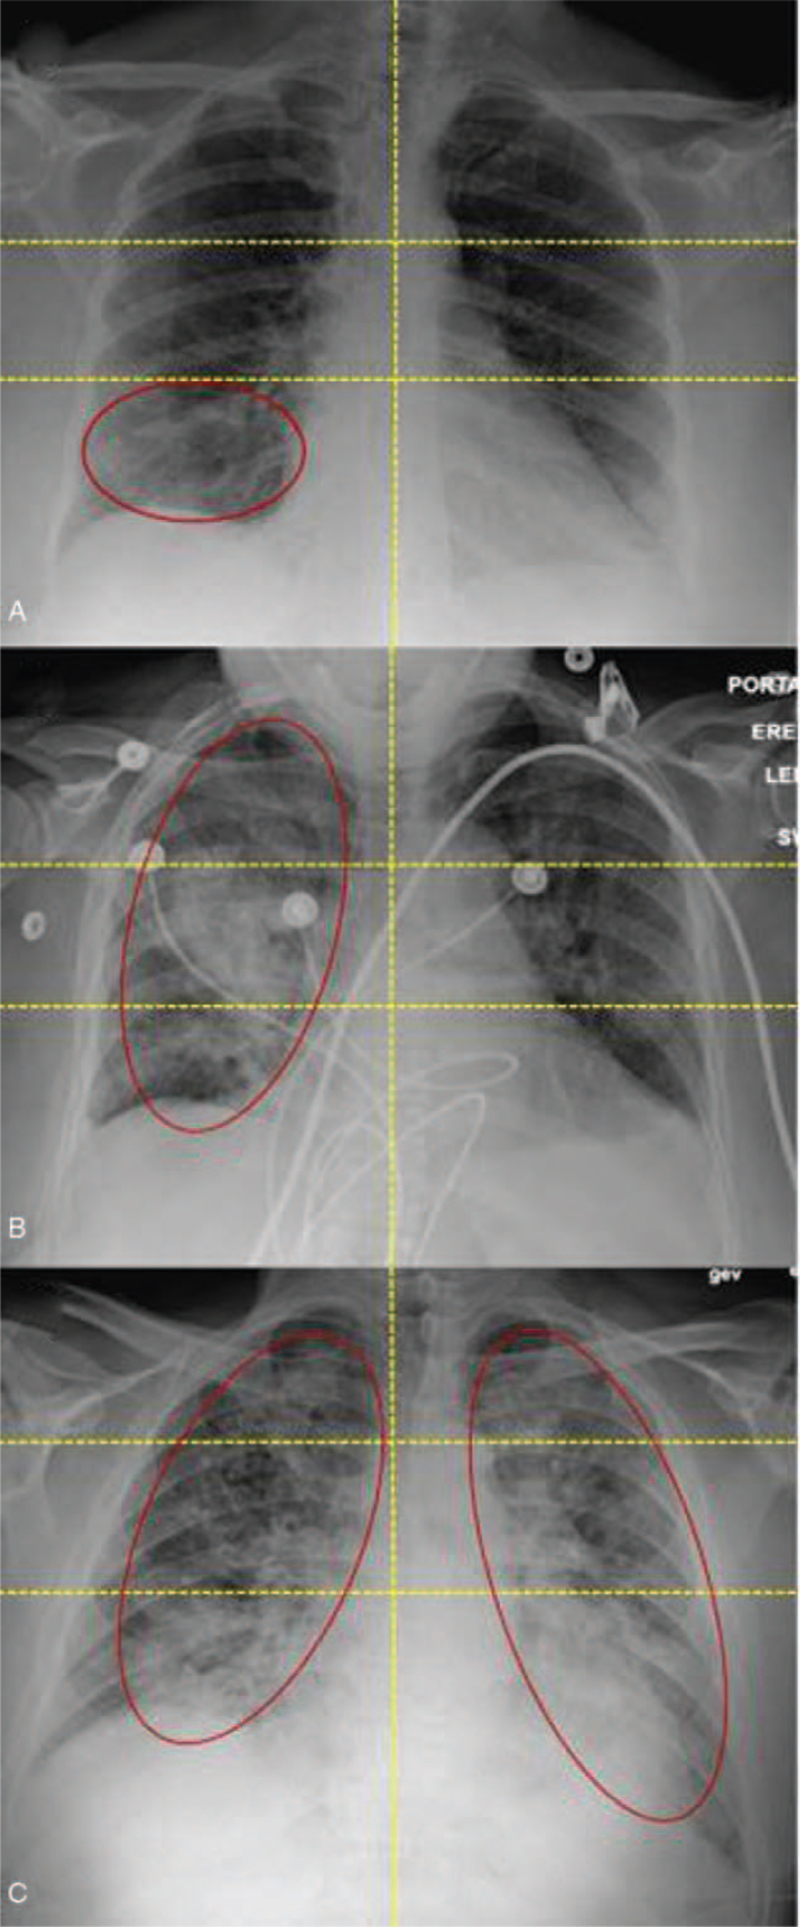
Examples of chest radiography (CXR) Sextus scores. (A) CXR of a 78-year old woman with a history of morbid obesity (BMI = 43), hypertension, and hyperlipidemia who presented with shortness of breath. Portable CXR shows left lower lobe opacity with a total Sextus score = 1 with opacities in the RLL (right lower lobe). (B) CXR of a 67-year old woman with a history of hypertension, cardiovascular disease, chronic kidney disease, chronic liver disease, and smoking who presented with dyspnea and shortness of breath. Portable CXR shows left lung opacities with a total Sextus score = 3 with opacities in the entire right lung. (C) CXR of a 61-year old overweight man (BMI = 27) with a history of hypertension and hyperlipidemia who presented with persistent dry cough. CXR shows diffuse lung opacities in bilateral lungs with a total Sextus score = 6. BMI = body mass index.
